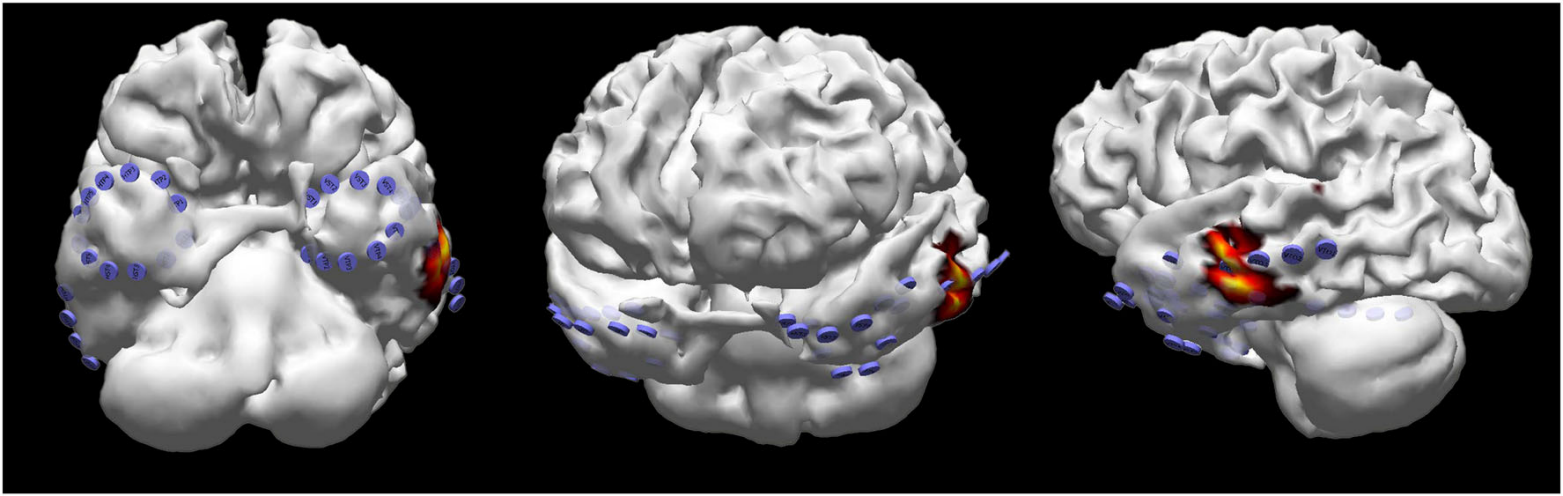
Location of the electrode contacts on superficial cortical anatomy of the temporal lobes, obtained by reconstruction of presurgical brain magnetic resonance imaging co-registered with computerised tomography scan after bilateral implantation of subdural strip electrodes, and localisation of the seizure onset zone on the laterobasal neocortex of the left temporal lobe, determined by the minimum norm solution of early ictal electroencephalographic activity, using methods as implemented in Curry software
